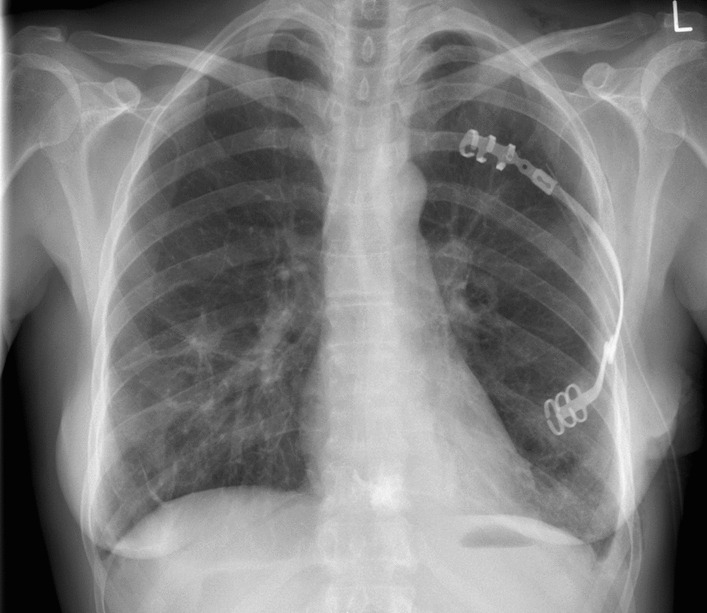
The postoperative X-ray provides a clear view of the implant's placement
